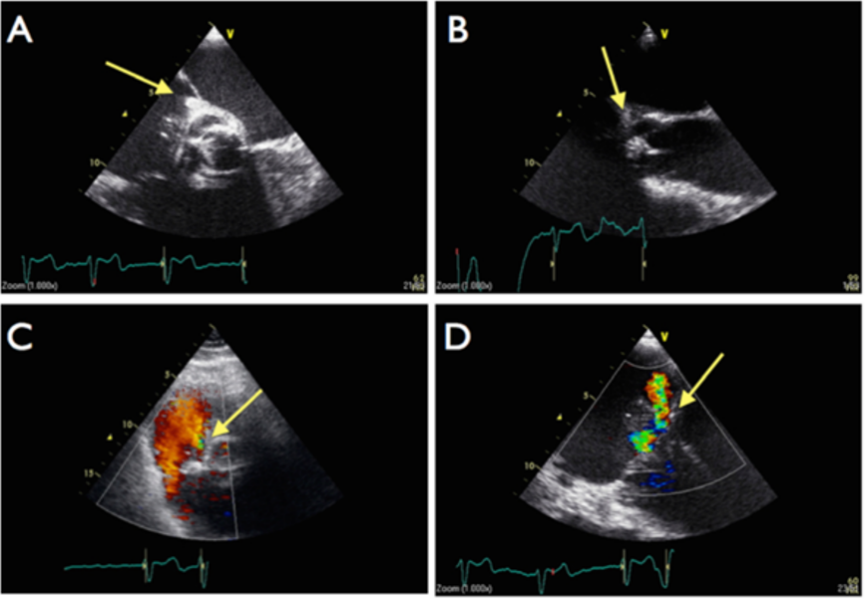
Transesophageal echocardiograpy views. Panel A: Mid-esophageal RV inflow/outflow view shows a paravalvular abcess near the atrial septum and involvment of the tricuspid valve insertion (arrow). Panel B: Mide-Esophageal long axis view showing vegetation on the aortic valve prosthesis. Panel C: zoomed in on the atrial septum, showing the Gerbode like defect with turblent flow (arrow). Panel D: severe tricuspid insufficiency (arrow).
